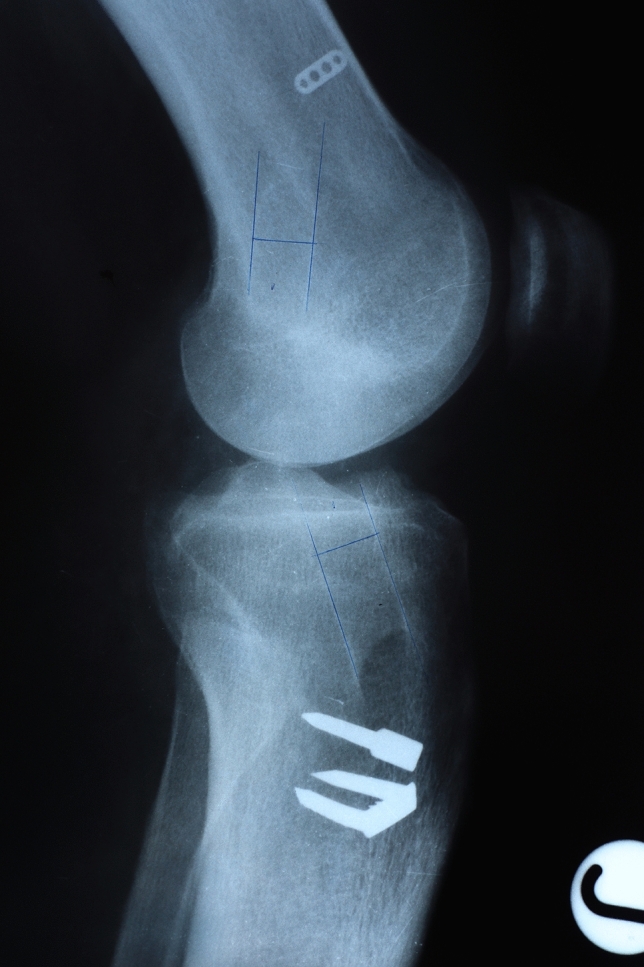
Tunnel widening on the lateral X-ray, using HT graft
